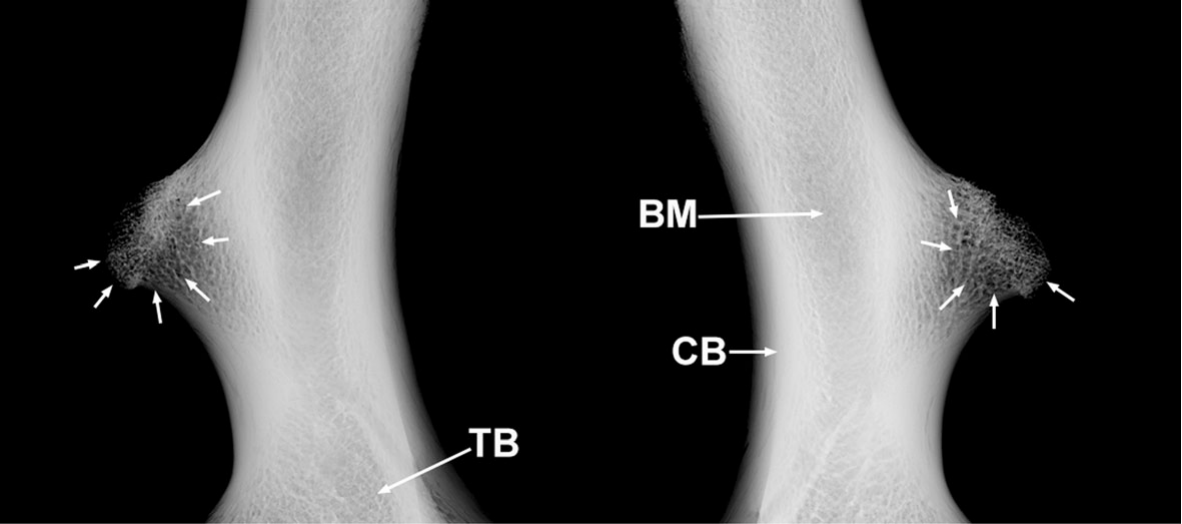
Radiographs of the injured humeri of *Tamandua tetradactyla* (MN 79028). BM, Bone marrow; CB, Cortical bone; TB, Trabecular bone. White arrows, radiolucent regions indicating bone resorption
